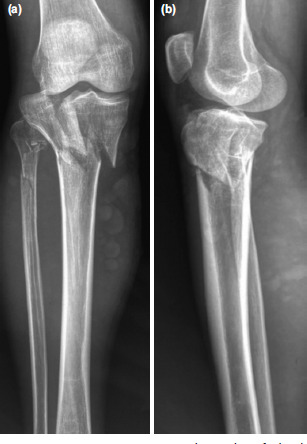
Case No.12, pre-operative radiographs of the knee showing Shatzker Type VI fracture of the proximal tibia (a). Anteroposterior view. (b) Lateral view.
